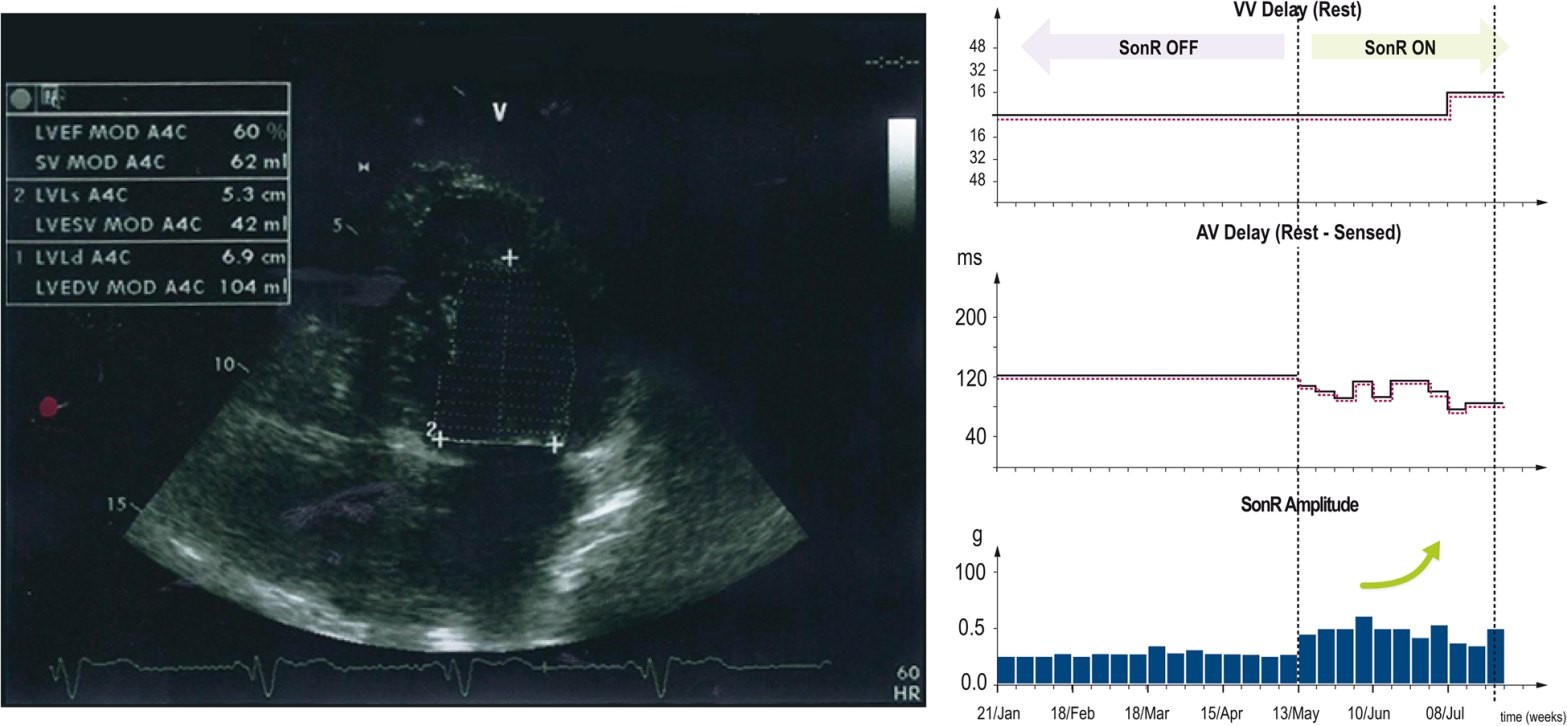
SonR parameters (interventricular delay at rest, sensed atrioventricular delay at rest, and SonR signal amplitude) before and after activation of the automatic optimization (in May 2013) and echocardiography
